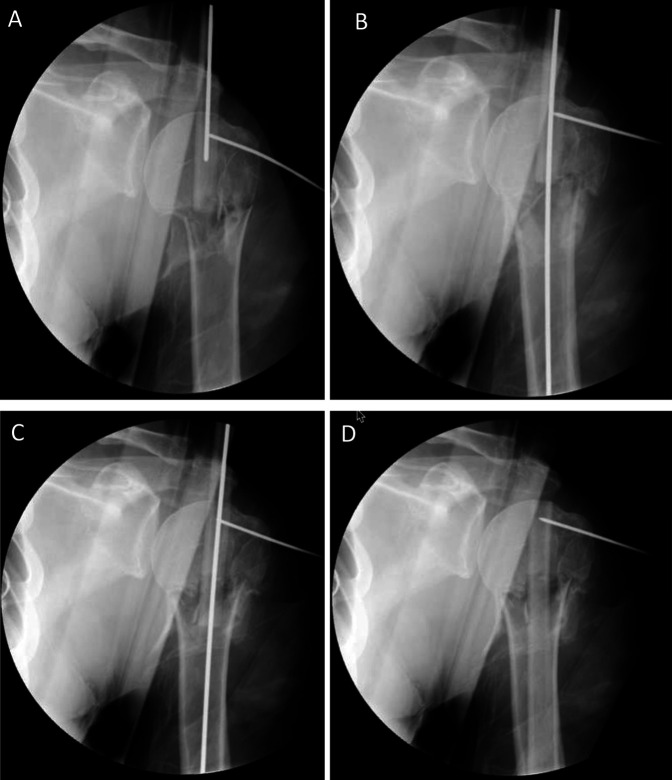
Radiograph demonstrating the cases where fracture site reduction is difficult, the blunt-end of a 2.5-mm ball-tipped wire may be passed through the medullary canal of the fibula (A) and into the medullary canal of the distal segment (B). The fibular strut may then be gently advanced into the distal segment (C and D).
